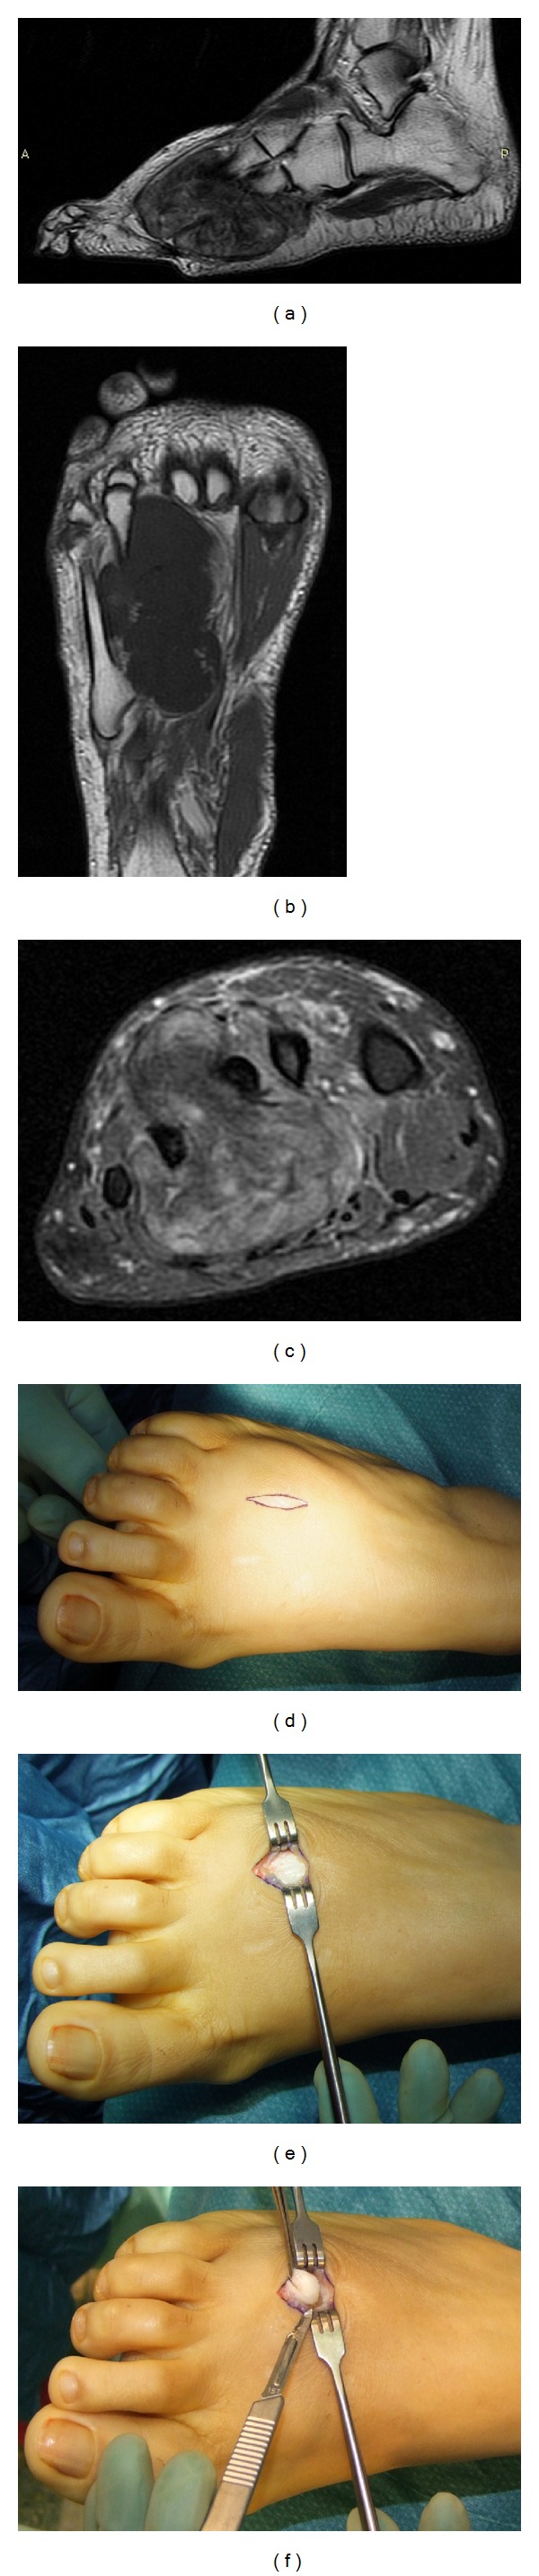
Sagittal MRI T2 (a), coronal MRI T1 (b), and axial MRI fat sat (c) scans of the right foot show heterogeneous intratumoral hypointensive signal on T1 and hyperintensive signal on T2. Incisional biopsy is made by (d) a small longitudinal incision between the second and third metatarsals; then (e) once the tumor capsule is found, (f) the tissue sampling is done with cold knife. Diagnosis: soft tissue chondroma.
